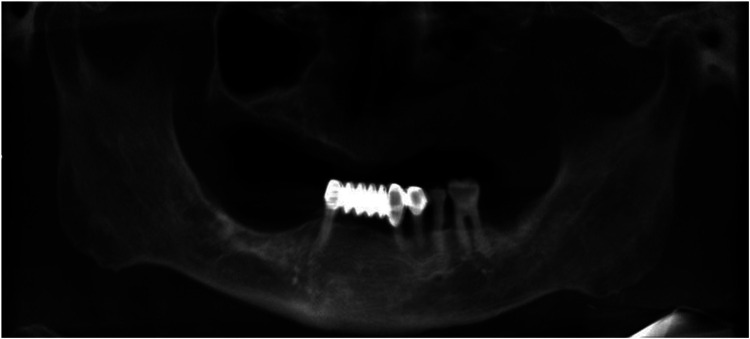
Initial cone-beam computed tomography image.
